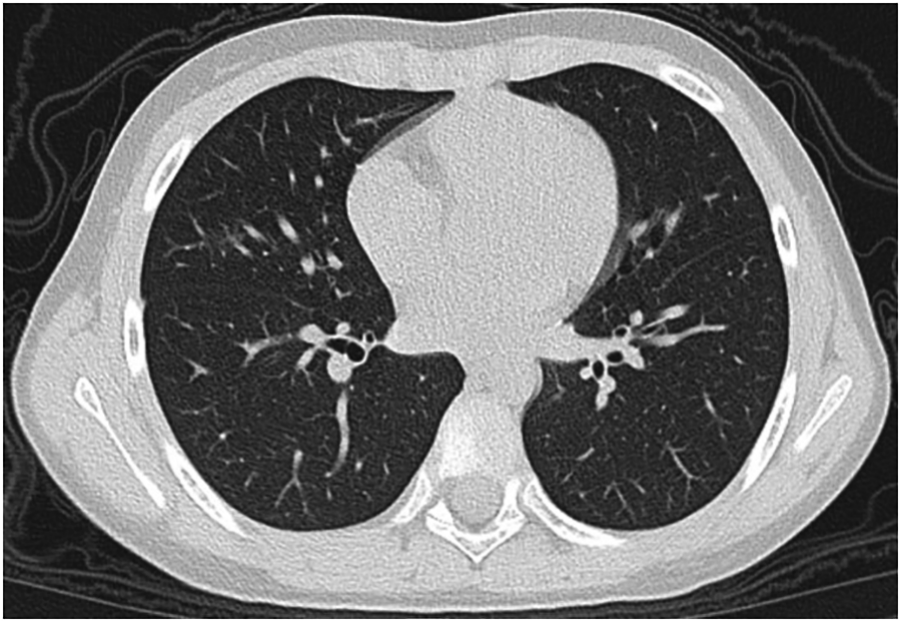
8-year-old boy infected with the Omicron variant of SARS-CoV-2. Unenhanced chest CT scans show ground glass opacity in the right middle lobe. CT findings were assigned CT severity score of 1.
